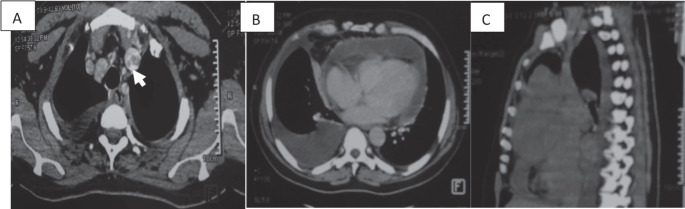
CT scan of a 58-year-old man (a): Cross-section of brachiocephalic trunk thrombosis (white arrow). (b): Cross-section of right pericardial and pleural effusion. (c): Sagittal section with a mediastinal mass.
